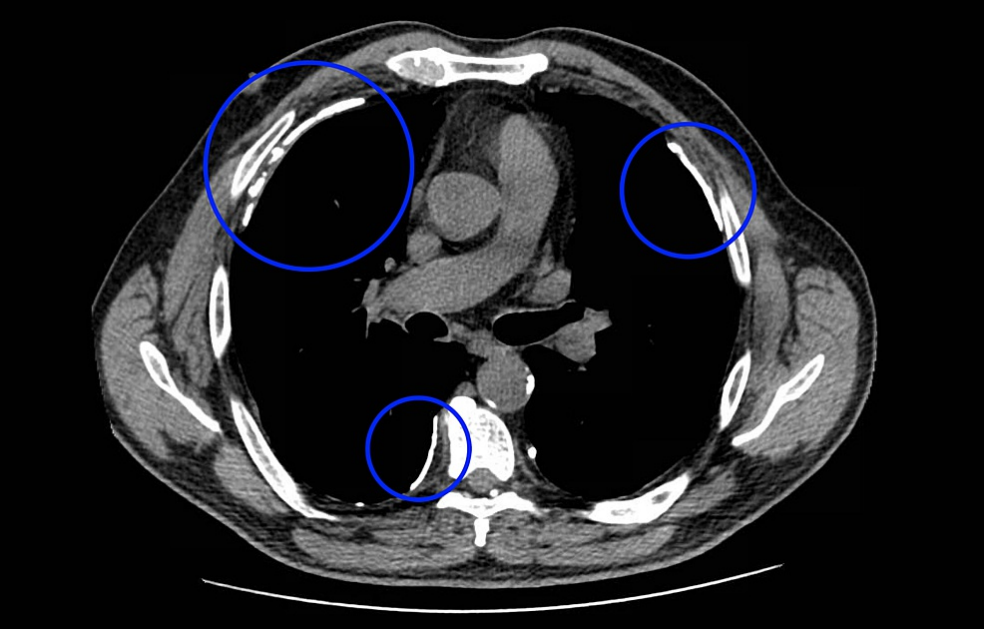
Thorax CT scan: pleural plaques, surrounded by blue circles, as signs of asbestos exposure
